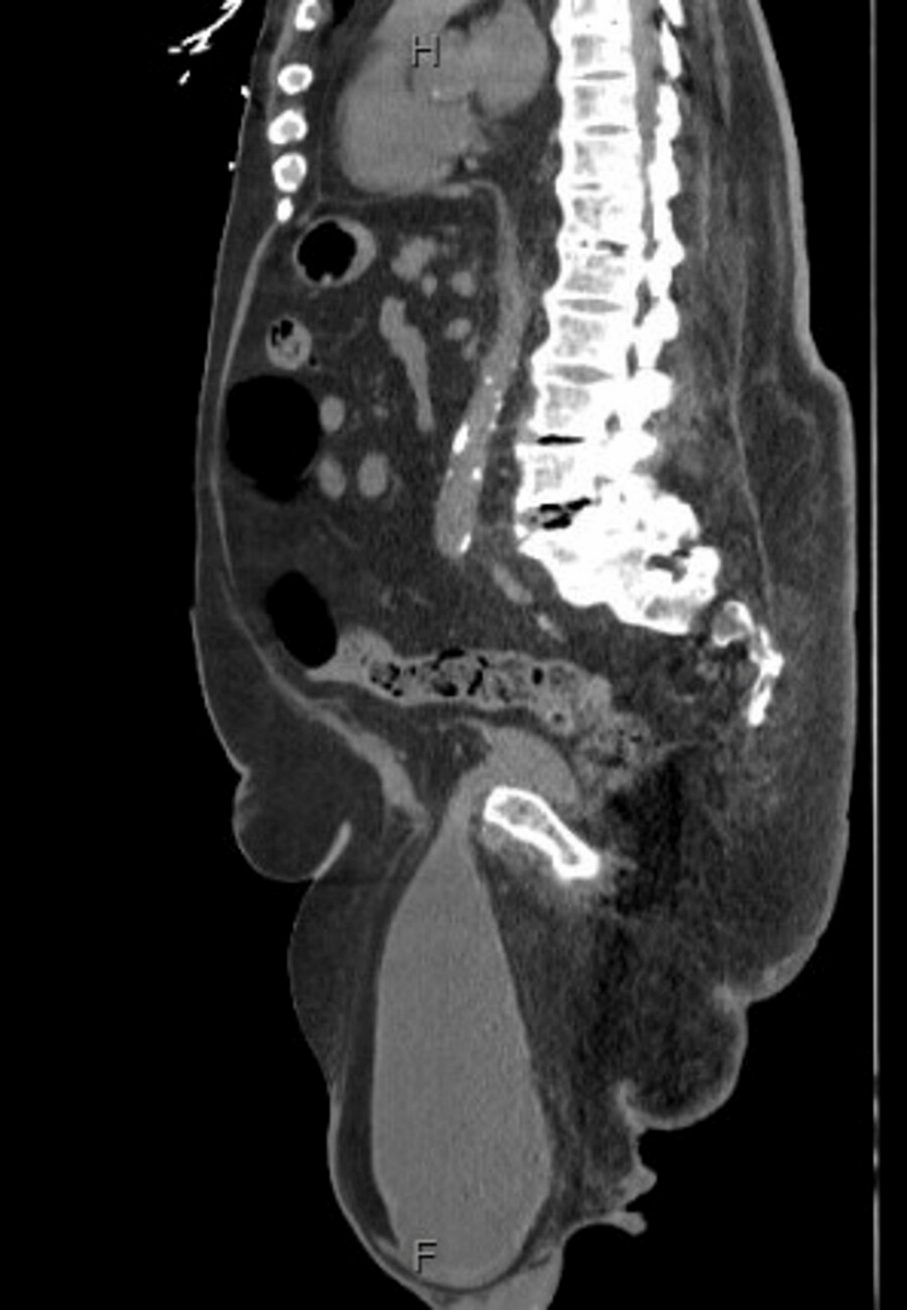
CT scan of abdomen and pelvis sagittal view showing a large right inguinal hernia containing most of the bladder and the appendix CT: Computed tomography
